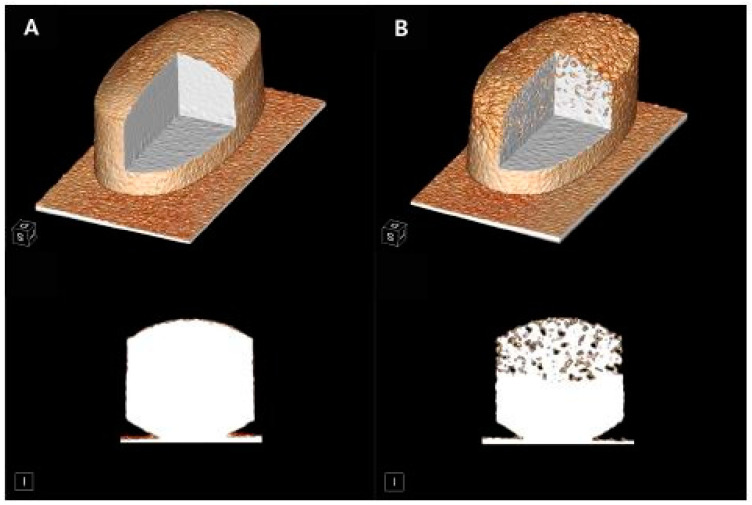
Images from 3D X-ray microcomputed tomography showing the non-porous tablet structure before sublimation (A) and the highly porous structure of the gastroretentive layer of the tablets after sublimation (B). Reprinted from International Journal of Pharmaceutics Vol 572, Hwang et al., Swellable and porous bilayer tablet for gastroretentive drug delivery: Preparation and *in vitro*-*in vivo* evaluation, 118783 (Pages 1–13), Copyright 2019, with permission from Elsevier.
